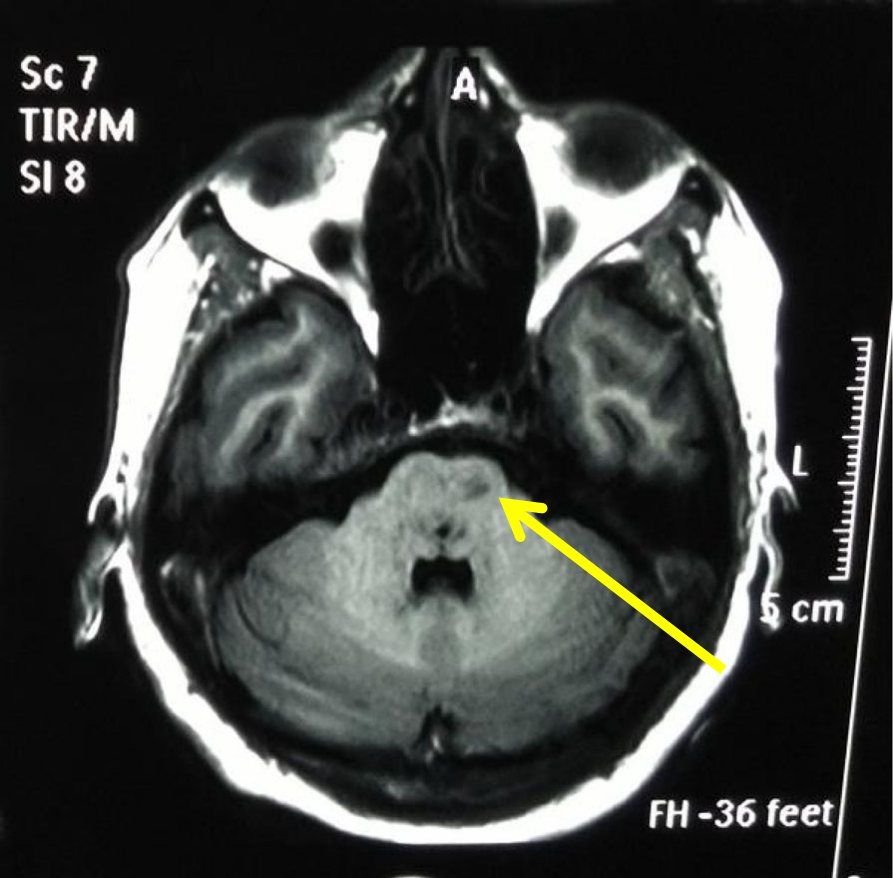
Magnetic resonance imaging scan of the brainstem showing multiple infarctions involving the pons and medulla regions (*arrow*)
